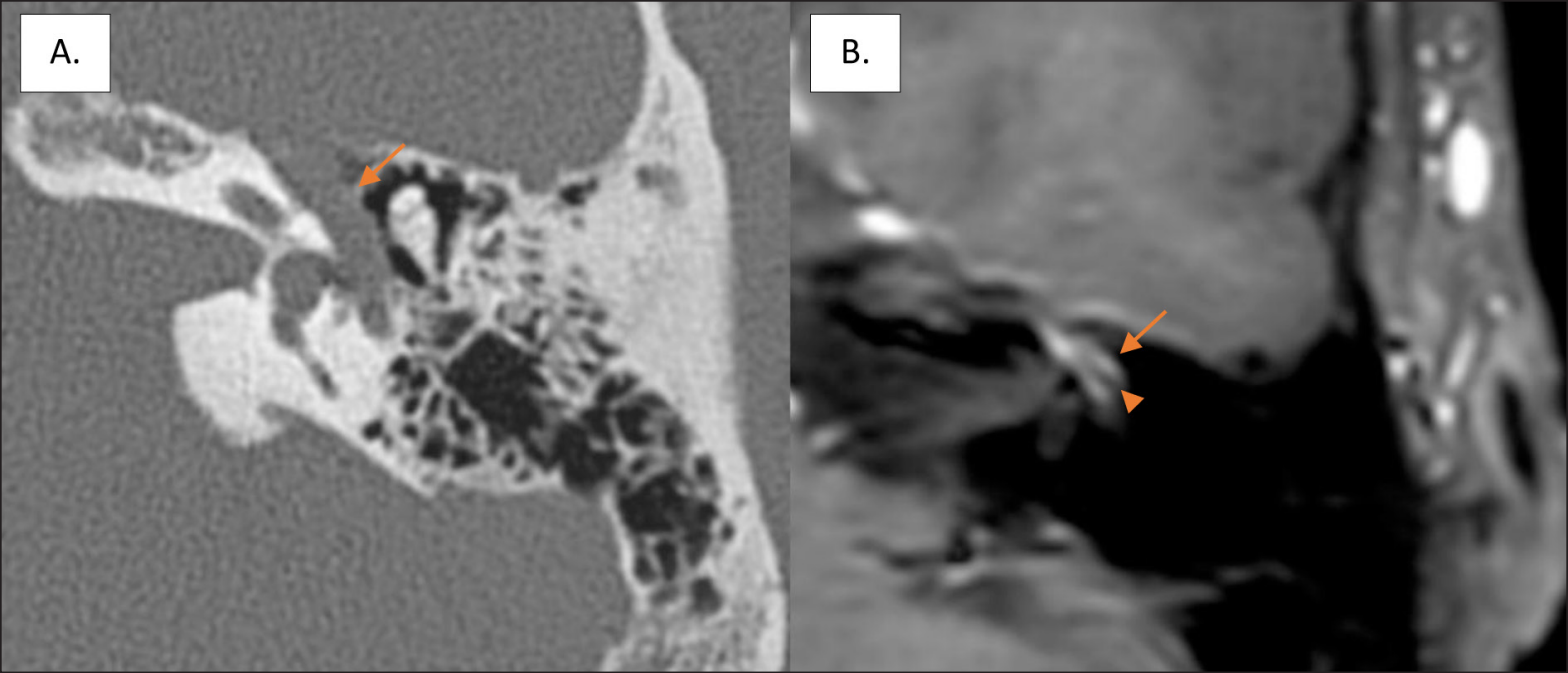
Enlargement of the tympanic segment of the fallopian canal (arrow) on axial CT scan of the left temporal bone (A) and intense contrast-enhancement (arrow) surrounding the facial nerve (arrowhead) on axial gadolinium-enhanced T1-weighted imaging (B).
